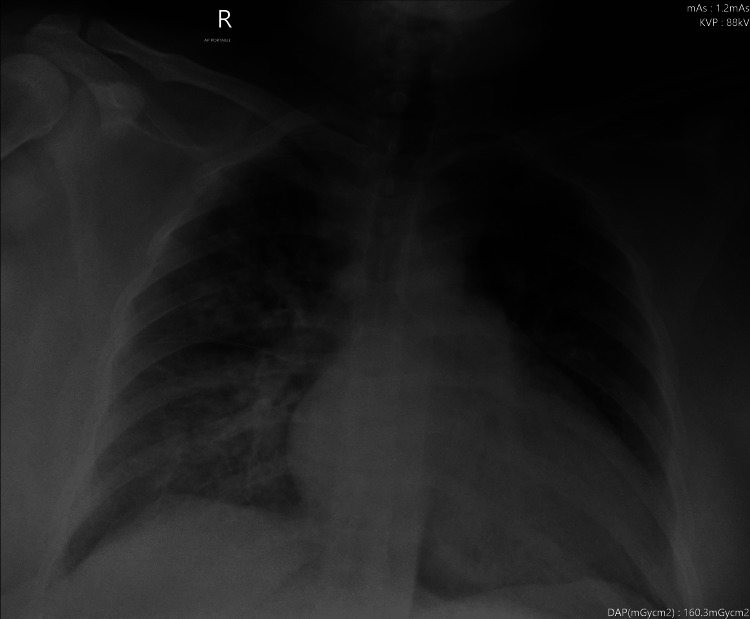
Chest X-ray on admission showing pulmonary edema
